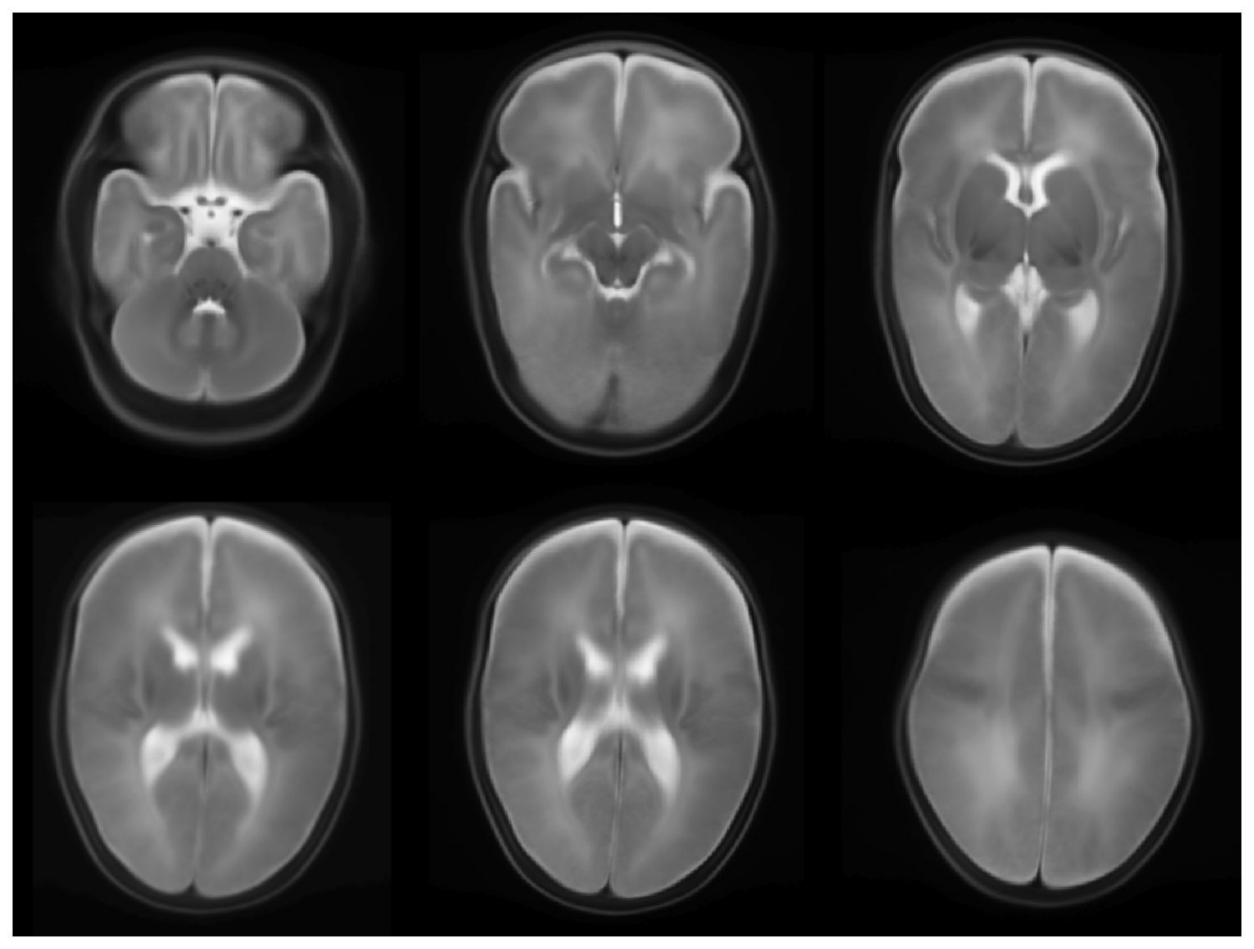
Several slices showing average brain image. This was achieved by iterative registration and summation of the T2-weighted images of all patients in the cohort.
